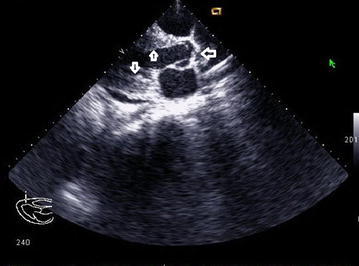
Echocardiography in parasternal long axis view showing supra-valvular aortic membrane causing SVAS in systolic phase (*horizontal arrowhead*) and increased left ventricular wall thickness (*vertical arrowhead*)
